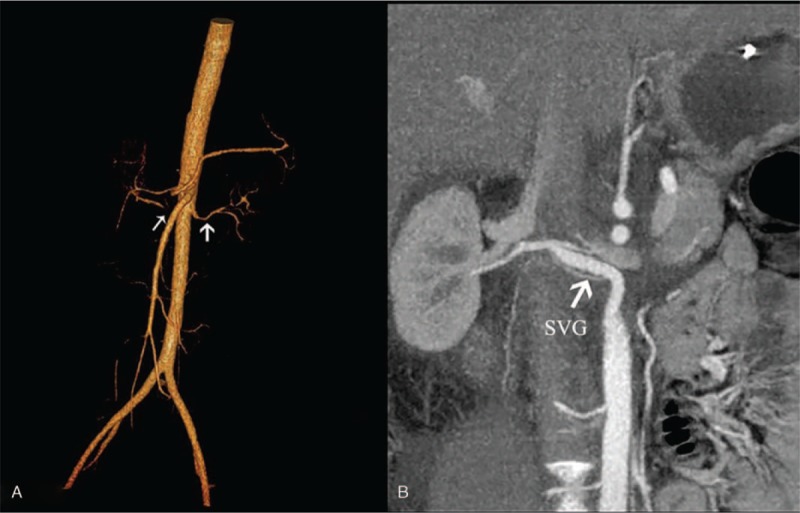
(A) CTA: Thin white arrow on the left indicates a severe stenosis lesion (>70%) in the middle segment of the right renal artery; thick white arrow on the right denotes a slender left renal artery. (B) CTA: Aortorenal bypass with SVG.
